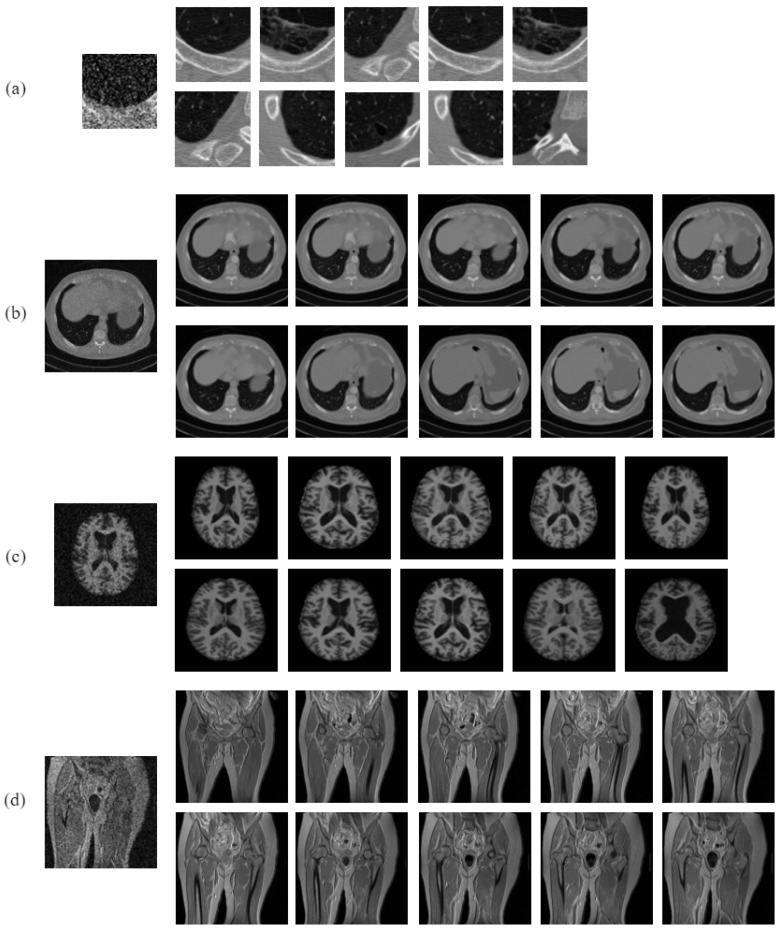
Query results of the proposed method for noisy query image on (a) Emphysema CT database, (b) NEMA CT database, (c) OASIS MRI database, and (d) NEMA MRI database.
